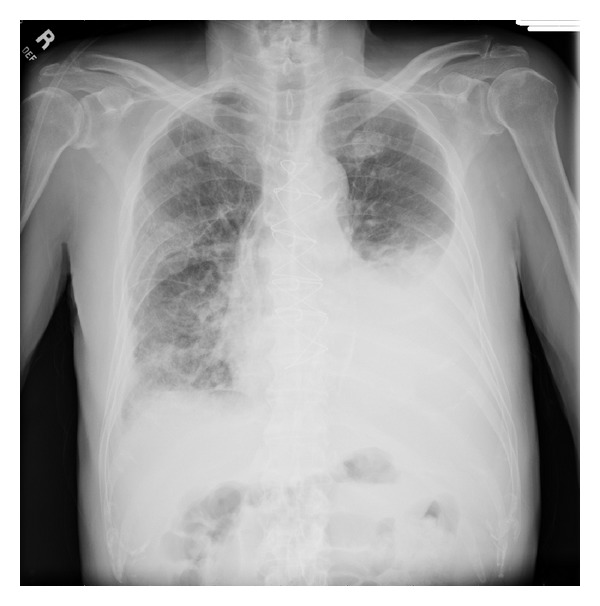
Chest radiograph showing moderate size left pleural effusion.
